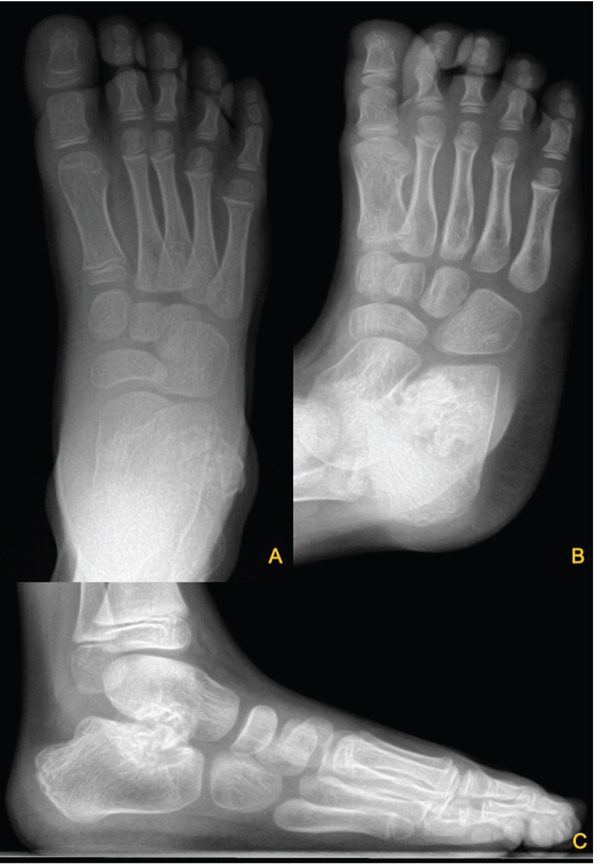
Radiographic images of the right foot including anteroposterior (A), oblique (B), and lateral (C) views demonstrating a pedunculated osseous lesion arising from the lateral talus.
